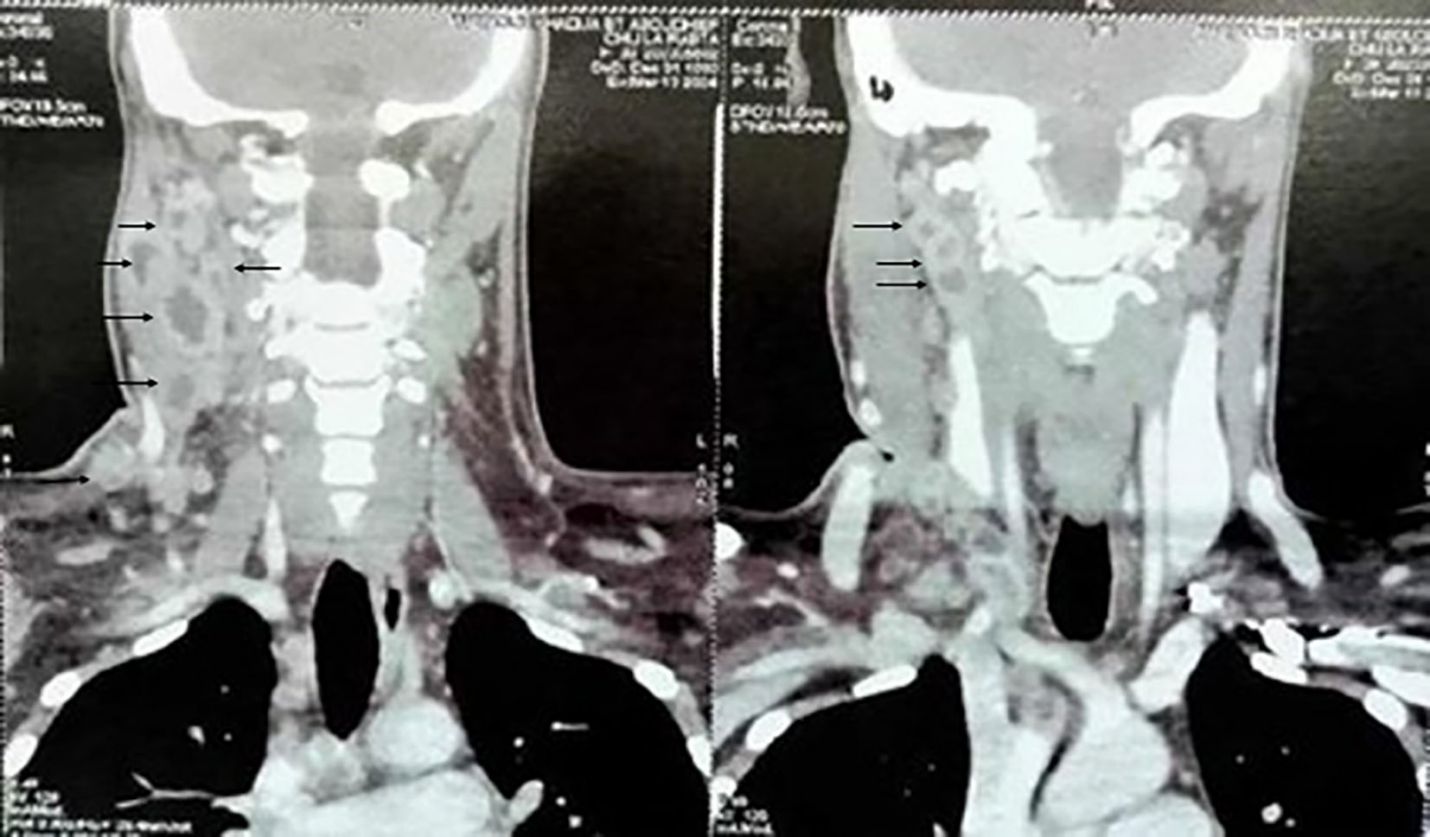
Multiple Necrotic Cervical Lymphadenopathies on CT Scan.
